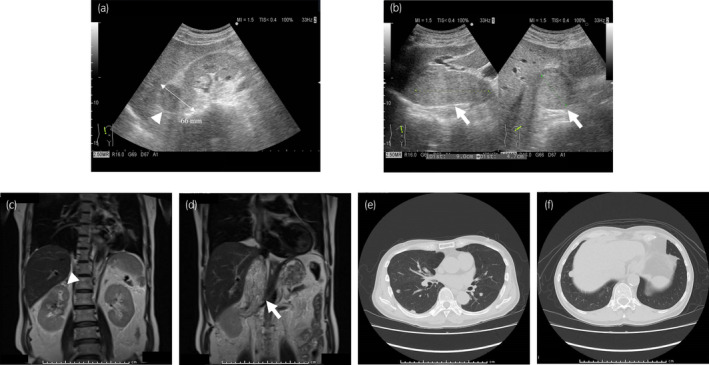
Images of the renal tumor in the upper portion of the right kidney and IVCTT. (a, b) Ultrasonography images. Ultrasonography revealed a 66 mm renal mass in the upper portion of the right kidney and a tumor thrombus within the IVC, the major axis and diameter of which is 90 and 47 mm, respectively. (c, d) T2‐weighted coronary images of MRI. MRI showed a renal mass at the upper pole of the right kidney and a tumor thrombus that extended into the IVC and beyond the hepatic vein. (e, f) CT images. CT revealed multiple pulmonary masses suspected of being lung metastases. [Colour figure can be viewed at wileyonlinelibrary.com]
